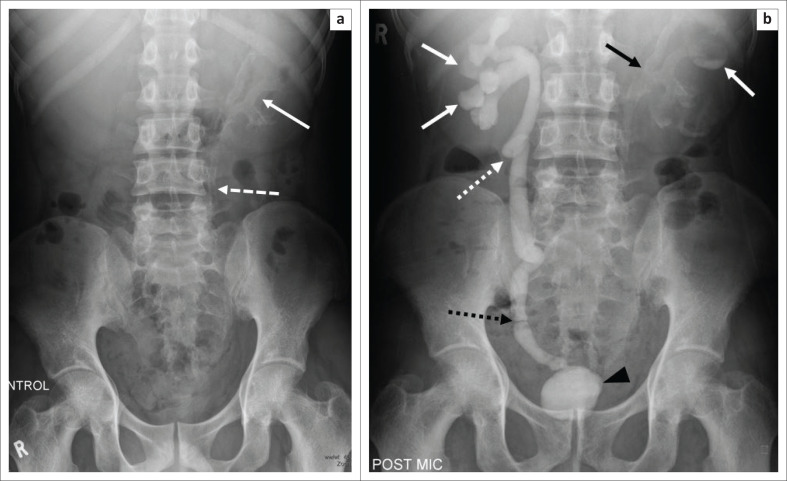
(a) A 43-year-old man known with human immunodeficiency virus and pulmonary tuberculosis, on both antiretroviral and tuberculosis treatment presented with a 3-month history of dysuria and right epididymal swelling. At intravenous pyelography, the control radiograph demonstrated diffuse urothelial calcification of the left pelvi-calyceal system (solid white arrow) and proximal left ureter (white arrow with dashed line). (b) A post-micturition view during intravenous pyelography in the same patient demonstrated bilateral blunting of the calyces (solid white arrows), a Kerr’s kink (white arrow with dotted line), focal stenosis in the distal right ureter (black arrow with dotted line), a small calibre bladder which contains residual contrast on the post-micturition view (black arrow head) and calcification of the left pelvi-calyceal system (solid black arrow).
